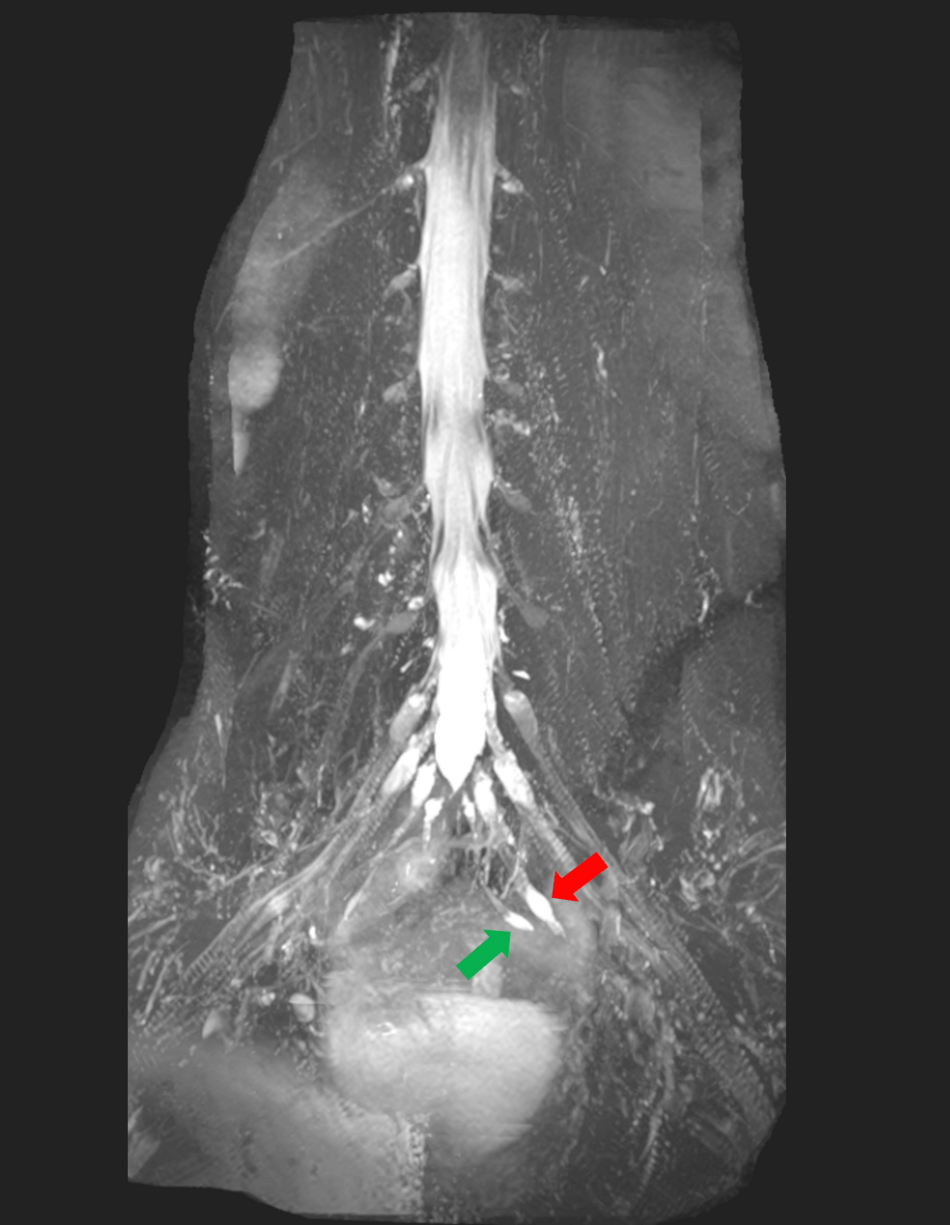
Nodular thickening and hyperintensity in the left-sided sacral plexus involving the left S3 (red arrow) and S4 (green arrow) nerve roots highlighted on maximum intensity projection of coronal view magnetic resonance imaging.
